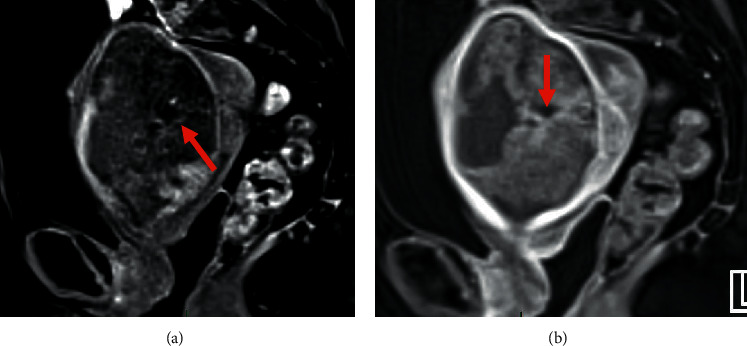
Pelvic MRI of MMMT: (a, b) sagittal DCE-MRI evaluated the presence of tumoral vessels in the lesion at arterial phase (arrow) (a) and percentage of necrosis at venous phase (arrow) (b).
